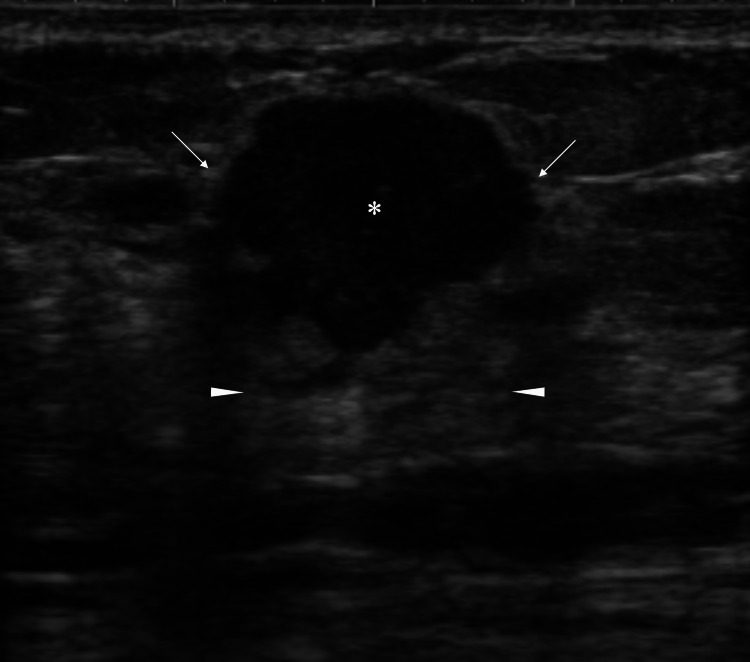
Ultrasound findings Ultrasound showed a polygonal tumor (asterisk) with internal low echoes, disruption of anterior borders of the mammary gland (arrows), enhanced posterior echoes (arrowheads),  and a tumor protrusion toward the overlying skin.
